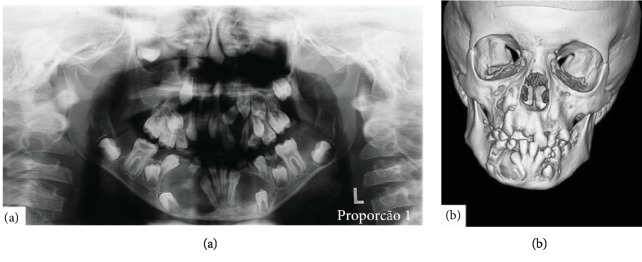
(a) Panoramic radiograph showing the multiple odontogenic keratocysts in the maxilla and mandible. (b) Computed tomography of the jaw.
